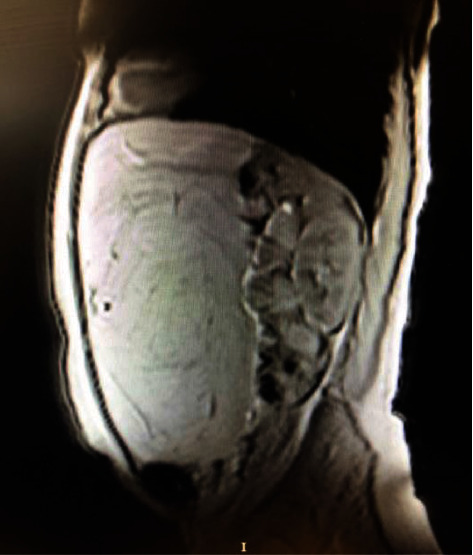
Sagittal view of magnetic resonance imaging shows that the lesion caused the intestines to move towards the posterior.
